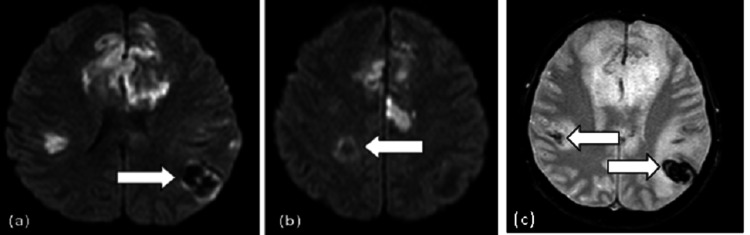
Axial non-enhanced MRI of the brain. Diffusion-weighted images show hypointense foci with a continuous rim of bright signal involving the left parietal (a) and right parietal lobes (b), consistent with hemorrhage (arrows). (c) SWI sequence demonstrates susceptibility artifacts in keeping with blood products MRI: magnetic resonance imaging, SWI: susceptibility weighted imaging
